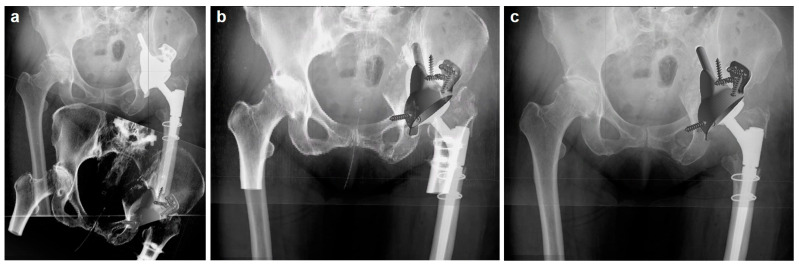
(a) Preoperative 3D CT-derived volume rendering of the pelvic bone with the planned implant position (bottom, gray implant), overlaid on the scaled 2D X-ray (top, white implant) to ensure dimensional accuracy. (b) Adjustment of the 3D CT volume-rendered pelvic model orientation to align with the X-ray, confirming anatomical landmark congruence while maintaining the implant’s planned position. (c) Final orientation adjustment with the pelvis fixed, showcasing the implant’s actual position in the X-ray for the postoperative analysis.
